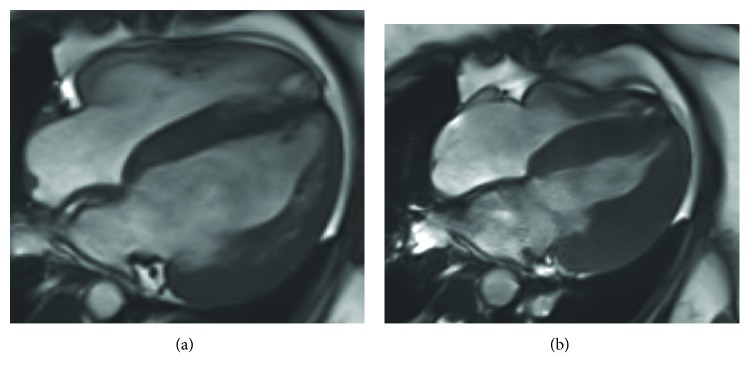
Cardiac MRI postcarnitine replacement in (a) diastole and (b) systole.
